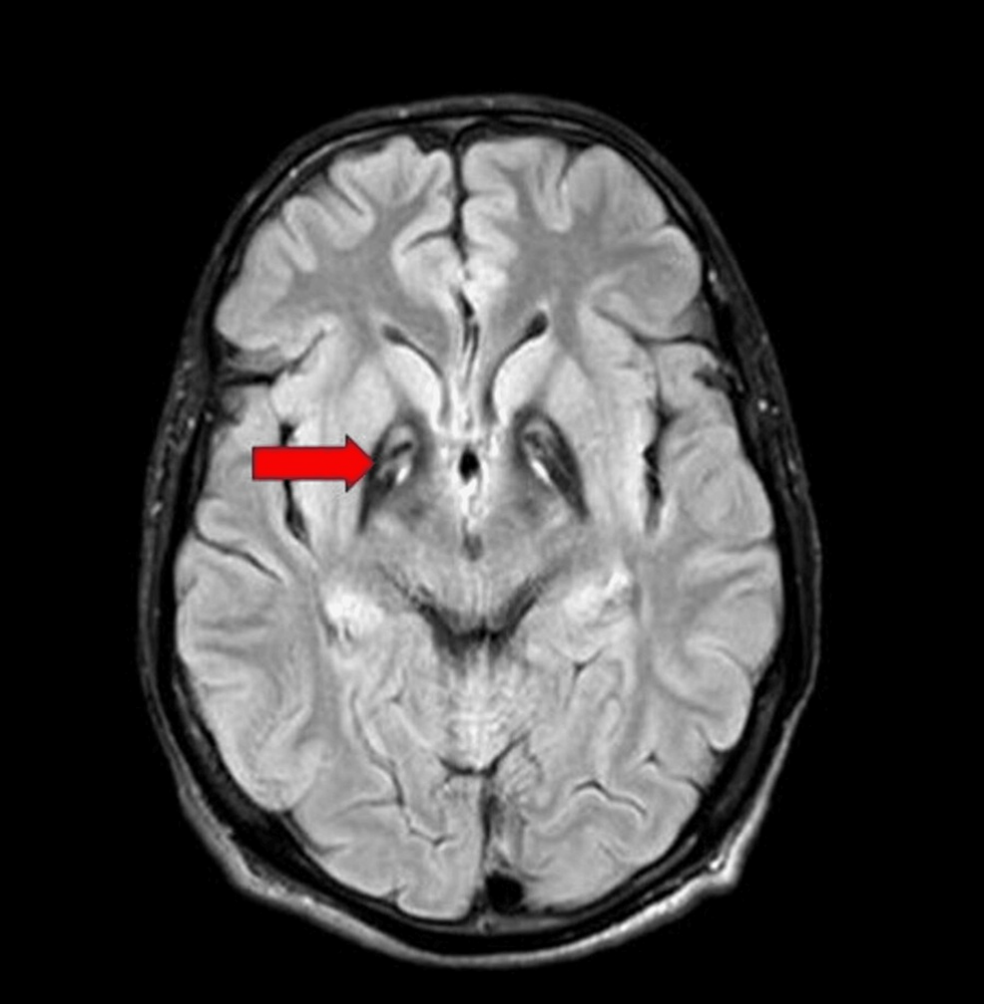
Brain MRI showing globus pallidus having bilateral and symmetrical hypodensities as well as the medial zone of hyperintensity with recognizable "eye of the tiger" sign (red arrow)
